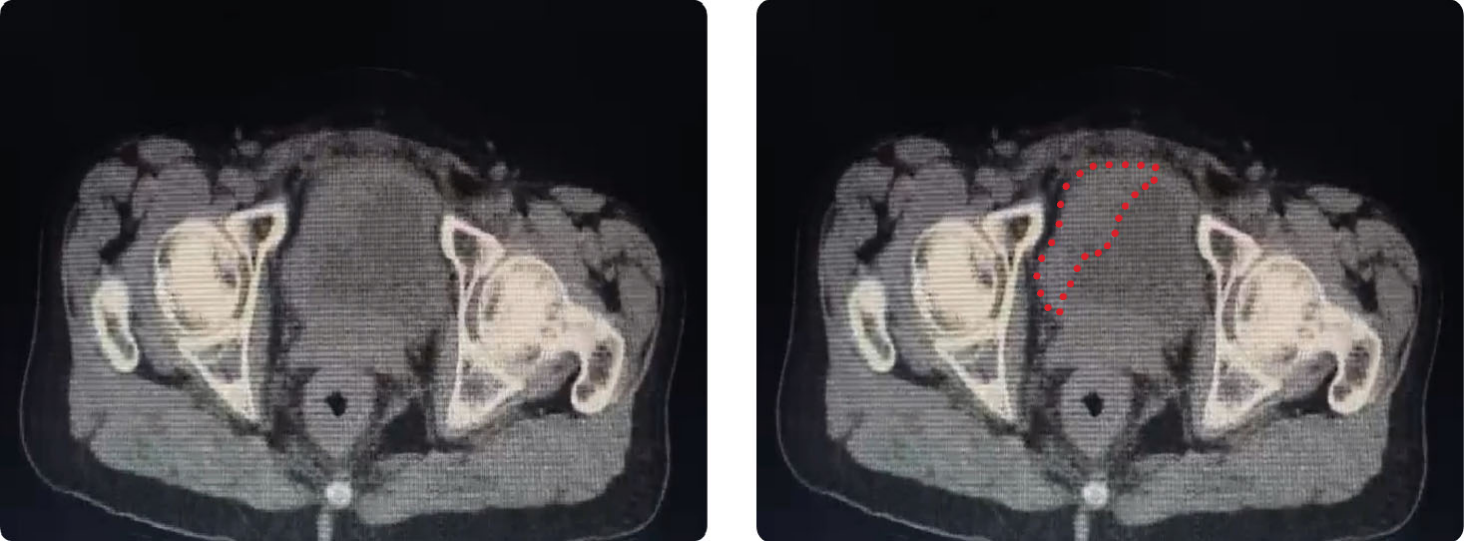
CT scan of the pelvis showing the bladder with the signet ring cell carcinoma mass (outlined in red dots) postoperatively upon 20 doses of immunotherapeutic treatment.
